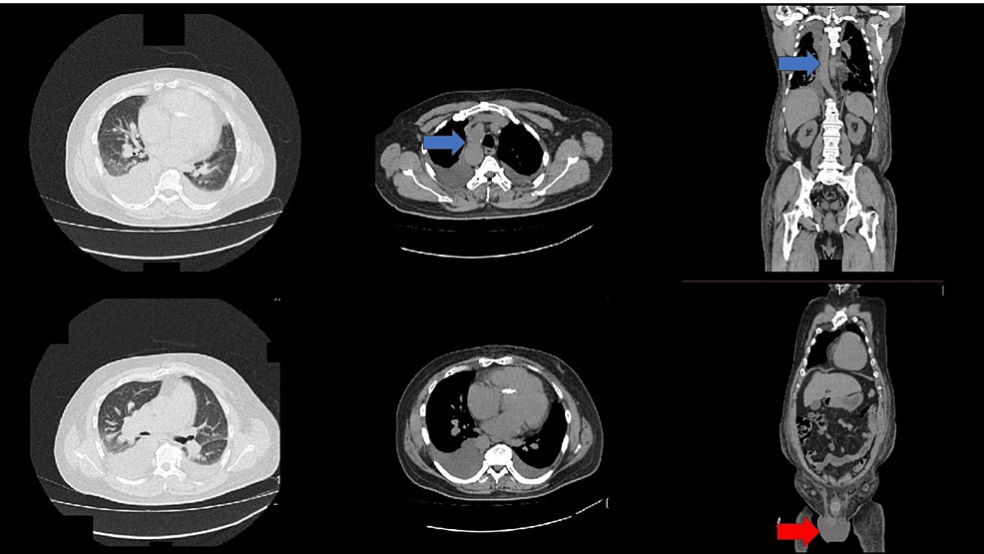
Chest and abdominal CT scan on admission Right-sided aortic arch (blue arrows), cardiomegaly, wall thickening of bronchi, pulmonary congestion, and hydrocele testis (red arrow) are observed.
